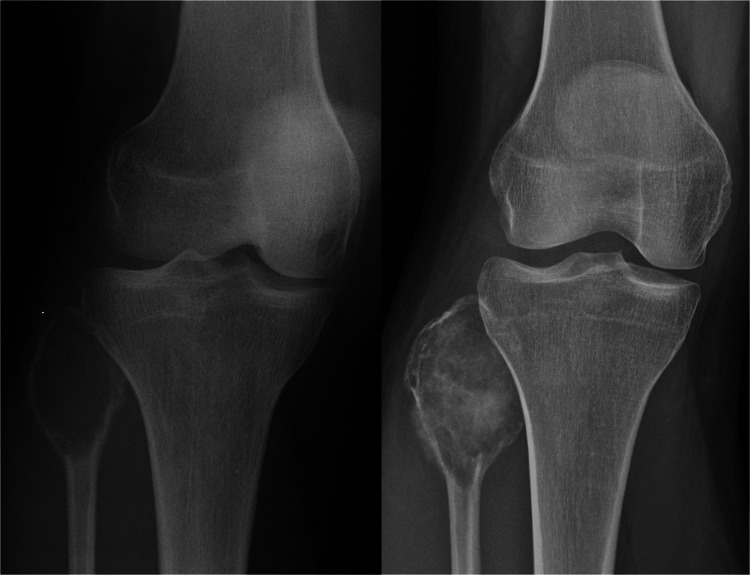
Fifty-five-year-old female with proximal fibular giant cell tumour and lung metastases (see Fig. S1). AP knee radiograph (left) reveals a markedly expansile lytic proximal fibular lesion with marked associated cortical attenuation. Thirty-seven-year-old female with proximal fibular malignant giant cell tumour with osteosarcomatous differentiation. AP knee radiograph (right) reveals an expansile proximal fibular lesion with sclerosis suggestive of matrix mineralisation (in keeping with the known osteosarcomatous differentiation) and a small ossified extraosseous mass laterally
